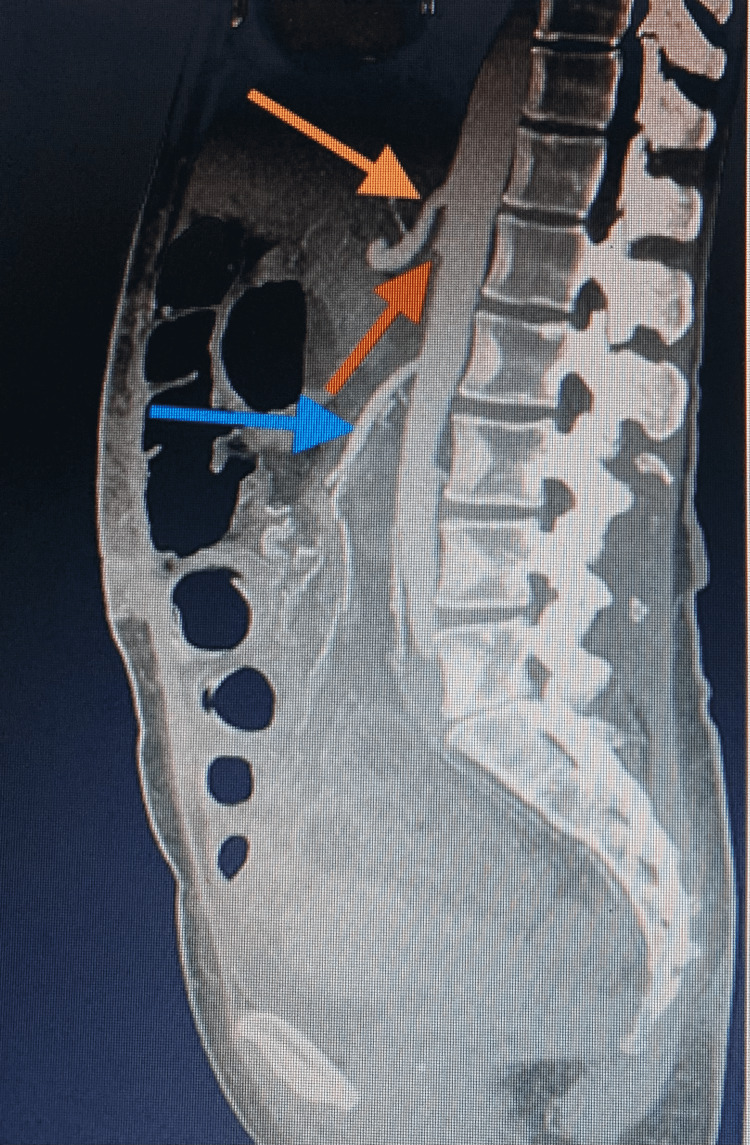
Control CTA scan. The superior mesenteric artery (blue arrow) after transposition with a normal aortomesenteric angle. Previous orifice of the superior mesenteric artery (red arrow) and celiac trunk (yellow arrow).
